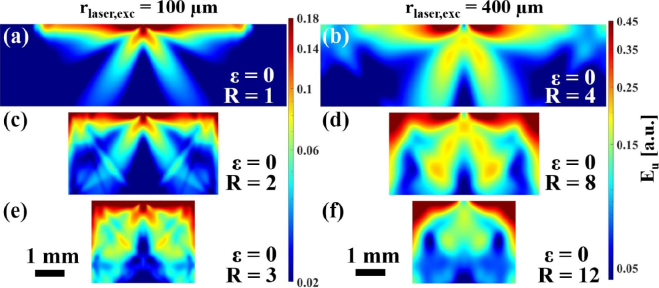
Ultrasound energy distribution $E_{u}$ in flat aluminum samples (ɛ = 0) in the thermoelastic excitation regime with central excitation (D = 0). In vertical direction the width of the samples was varied, in horizontal direction the absolute value of the radius of the excitation laser spot $r_{\mathrm{laser,exc}}$ leading to relative excitation laser spot sizes R between 1 and 12. A video of the wave propagation in (e) is provided in the Suppl. Mat. (Video S3).
